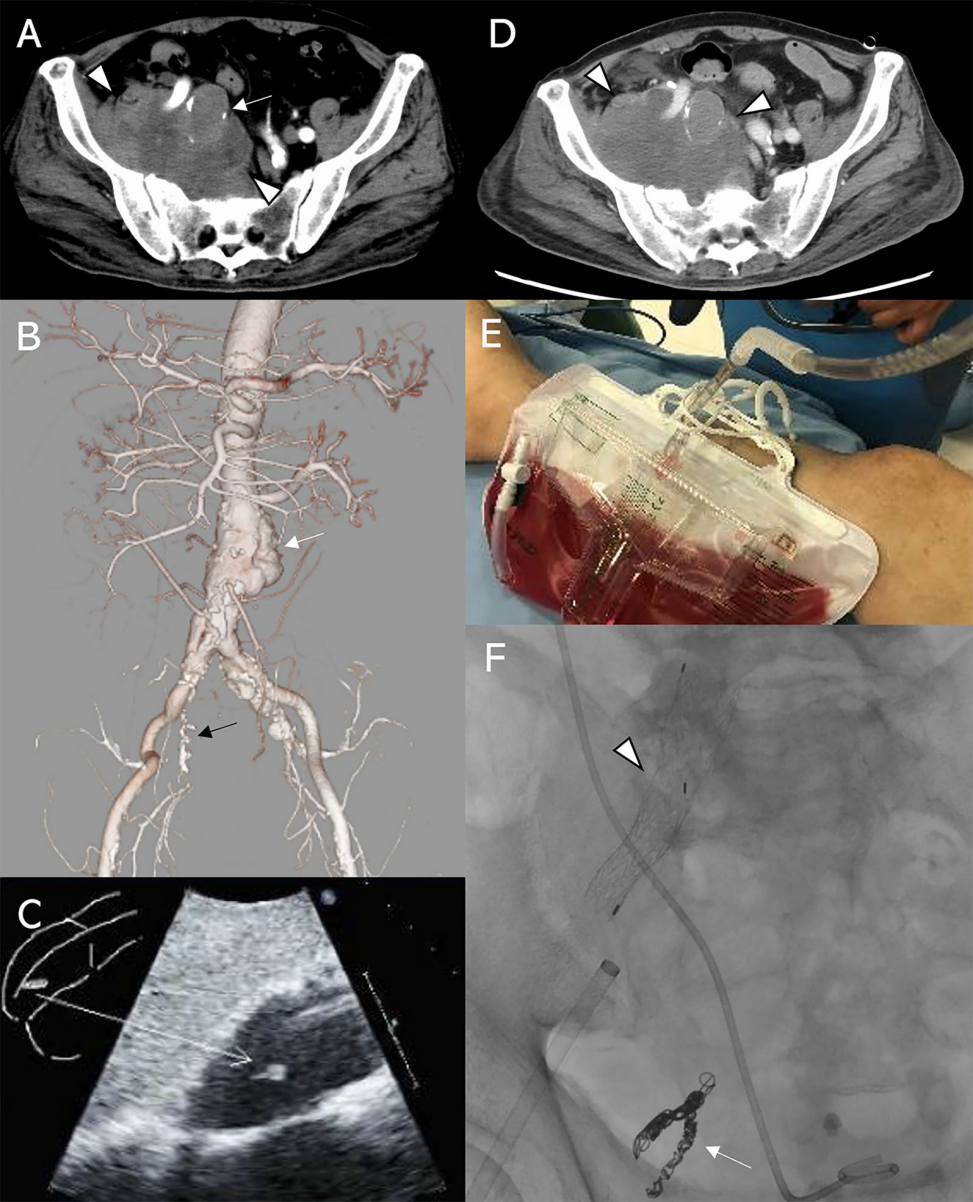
A Preoperative computed tomography (CT) revealed a 28-mm right internal iliac artery aneurysm (IIAA, arrow) and perianeurysmal fluid retention (arrowheads). B Three-dimensional CT angiography showed abdominal aortic aneurysm (white arrow) and occluded IIA (black arrow). C Mobile 15-mm mass was observed at the tip of the pacemaker lead as a source of infection. D CT revealed increased perianeurysmal fluid retention on day 18 of hospitalization (arrowheads). E Aggravated hematuria with increased perianeurysmal fluid retention. F Endovascular aortic aneurysm repair (arrowhead) and coil embolization (arrow)
